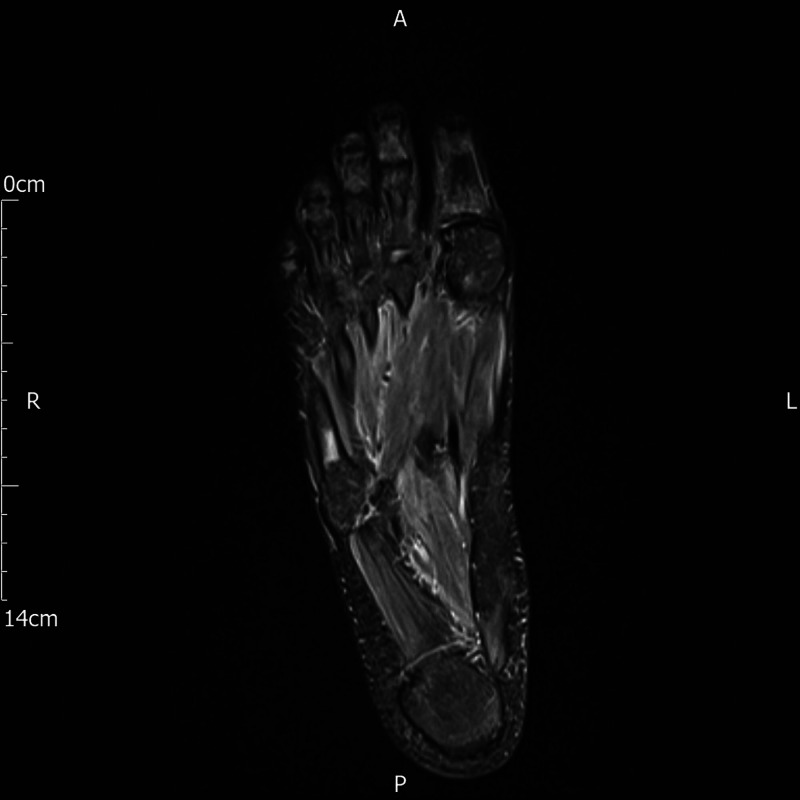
MRI right foot showing stress fracture in fifth metatarsal.
